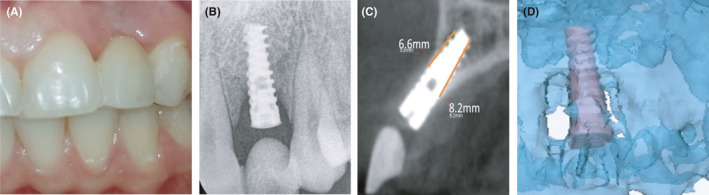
(A) Clinical photograph of implant site with a resin‐bonded bridge at 1‐year follow‐up. (B) Intraoral periapical radiograph of implant site at 1‐year follow‐up. (C) The CBCT imaging of implant site showing bone level in sagittal view. (D) 3D volume matching of implant site before treatment and 1 year after treatment shows new bone formation in mesial and distal sites of implant
